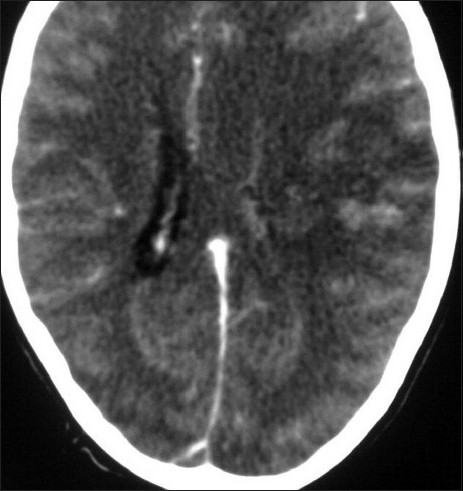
Contrast-enhanced transverse CT image showing the empty delta sign in the superior sagittal sinus posteriorly, with a large infarct in the lefthemisphere causing a midline shift to the right
